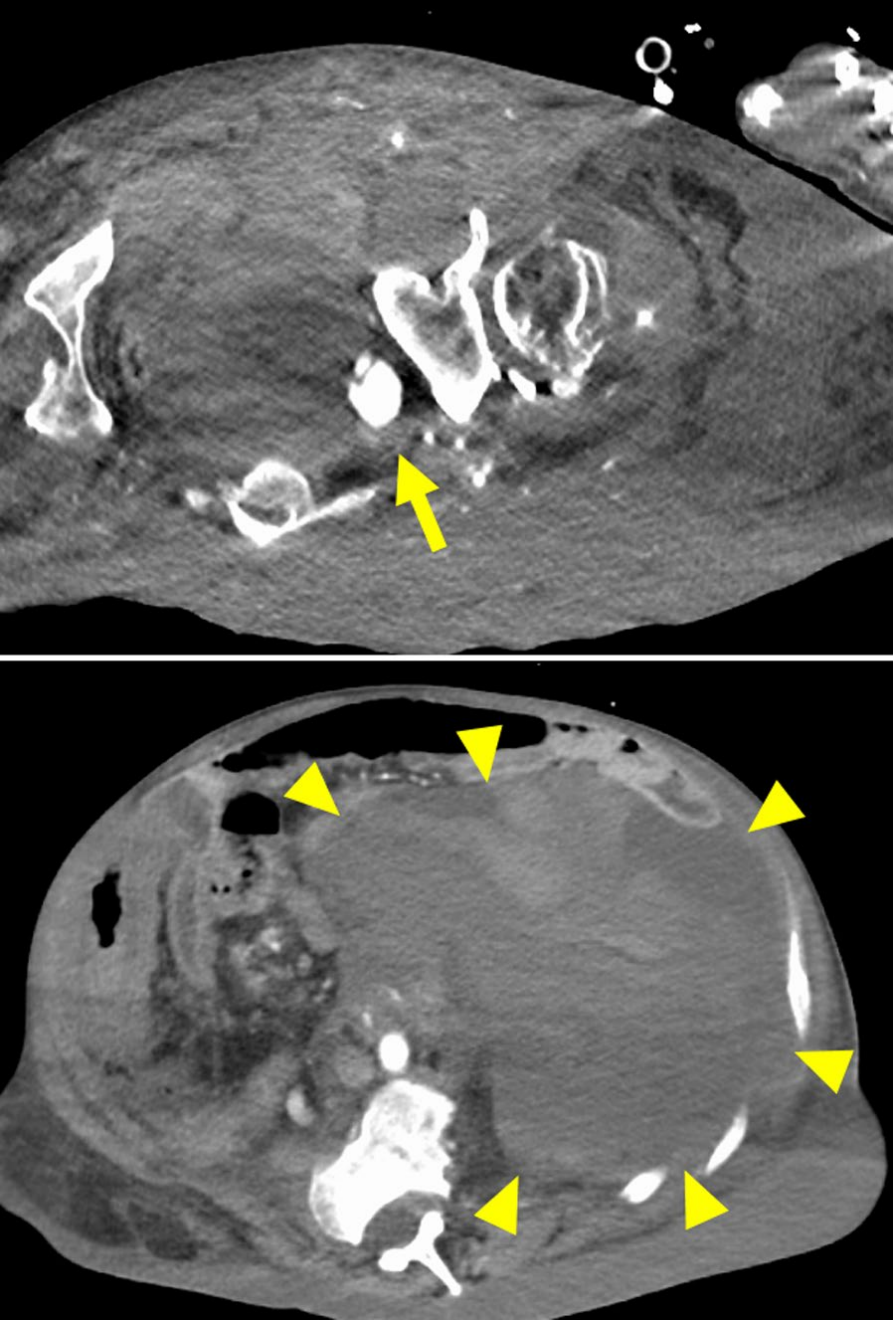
Top panel: Contrast-enhanced computed tomography revealed a 2-cm-diameter aneurysm of the left internal iliac artery (arrow). Bottom panel: Contrast-enhanced computed tomography revealed a huge retro peritoneum hematoma (arrowheads) compressing the intra-abdominal organs
